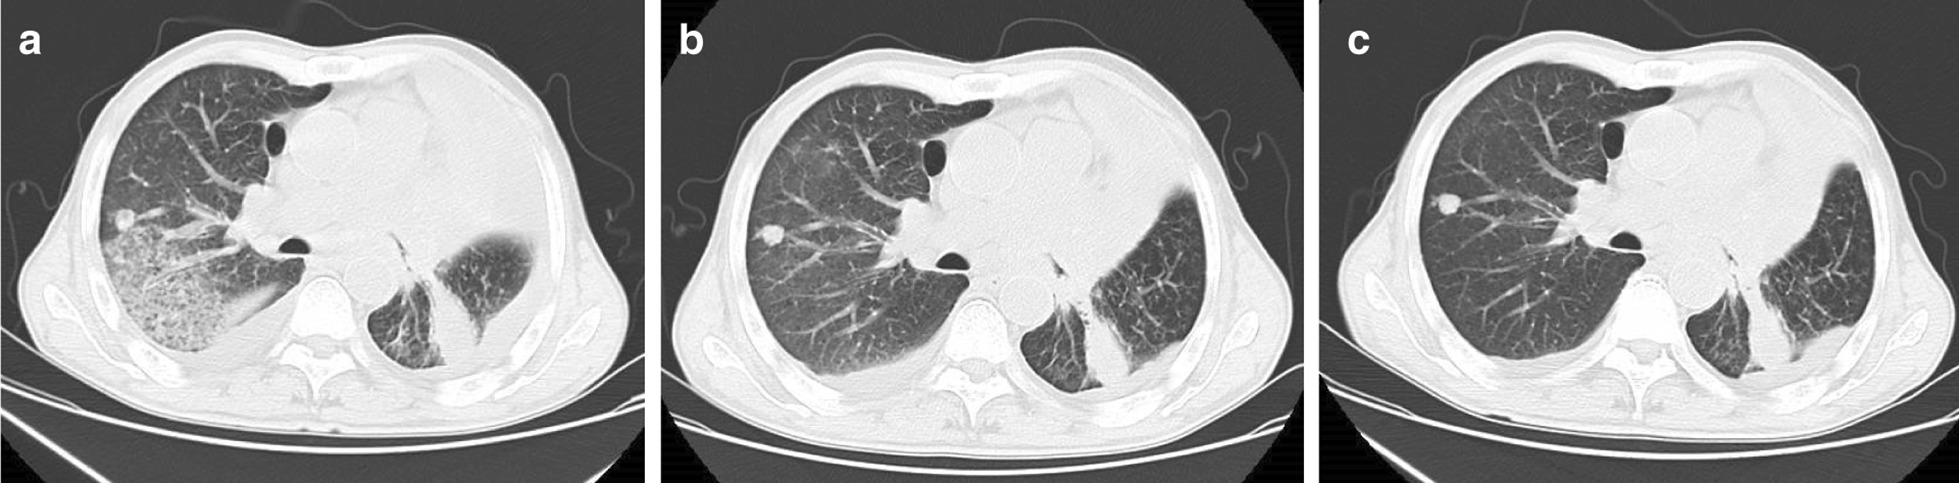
Assessment of the pneumonitis lesion via chest CT scan before (a) and after 3 days (b) and 7 days (c) of prednisolone and antibiotics treatment
